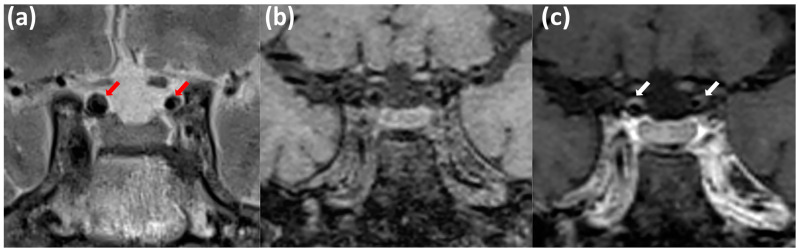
Patient ID#2. MRI signs of intracranial atherosclerosis of the brachiocephalic arteries: uneven stenosis of both internal carotid arteries (ICA) at the intracranial level, with signs of an eccentric pattern of contrast enhancement. MRI in T2 mode with high resolution (T2-HR) in the coronary (a), MRI T1 db f/s, before (b) and after (c) intravenous contrast injection with high-spatial-resolution T1-weighed imaging and suppression of the signal from bloodstream and fat. Red arrows—intracranial atherosclerotic plaque; white arrows—contrast enhancement in the vessel wall.
